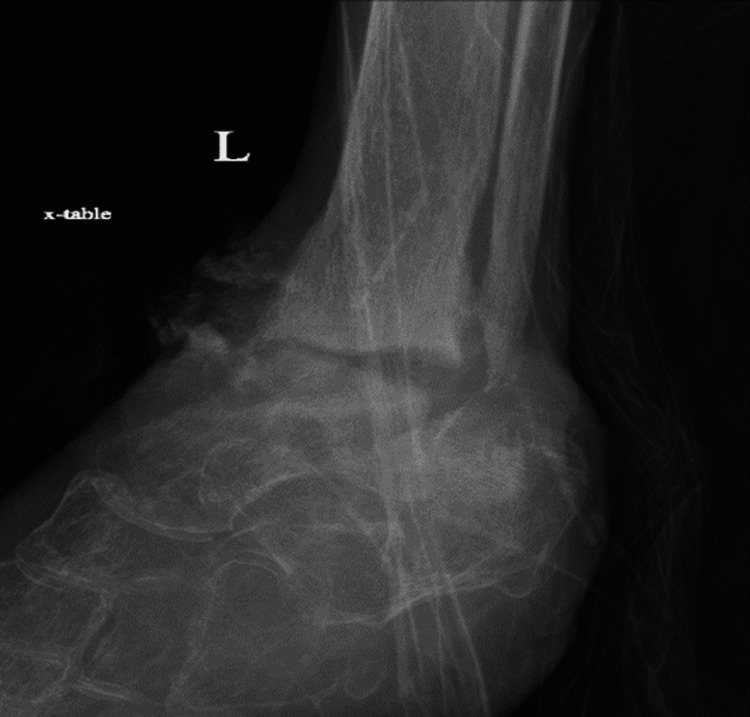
Mortise X-ray of the left ankle/foot
